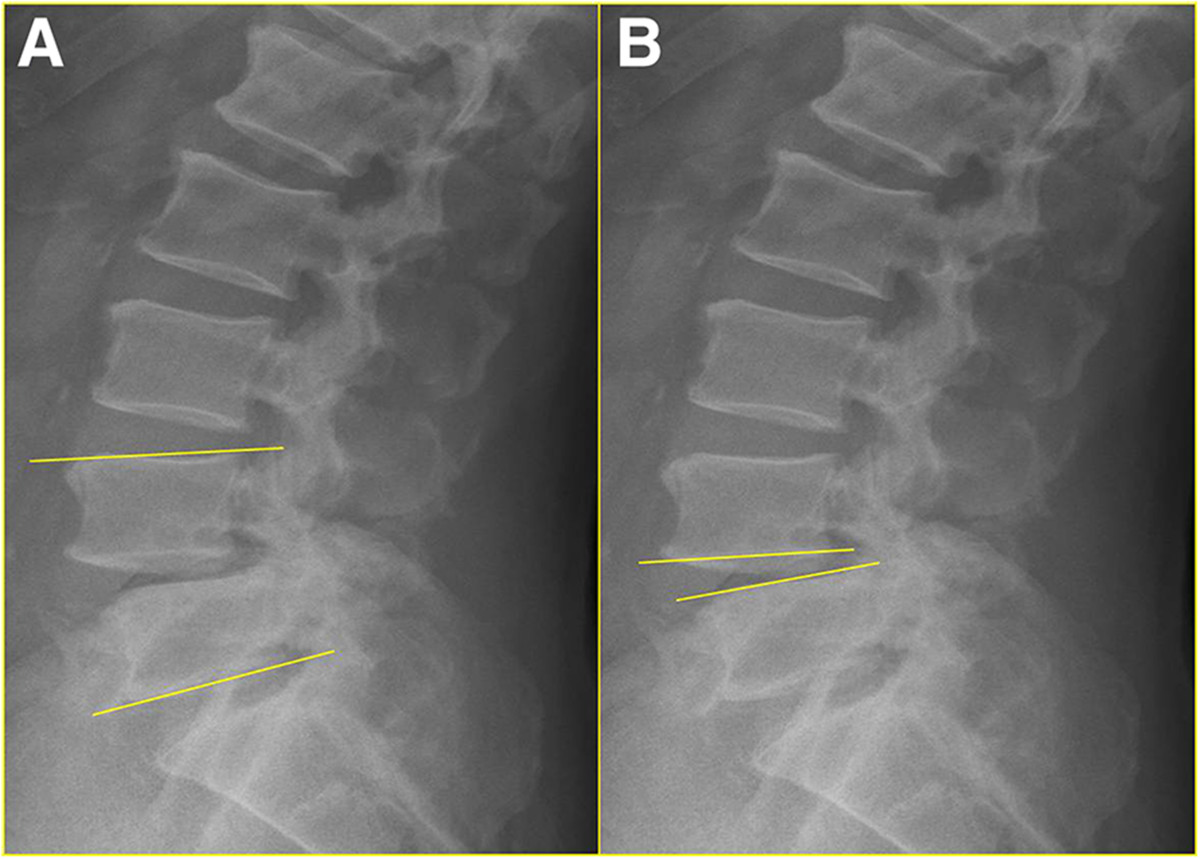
Preoperative lateral radiographs were used to measure segmental lordosis (A) and intradiscal lordosis (B).
